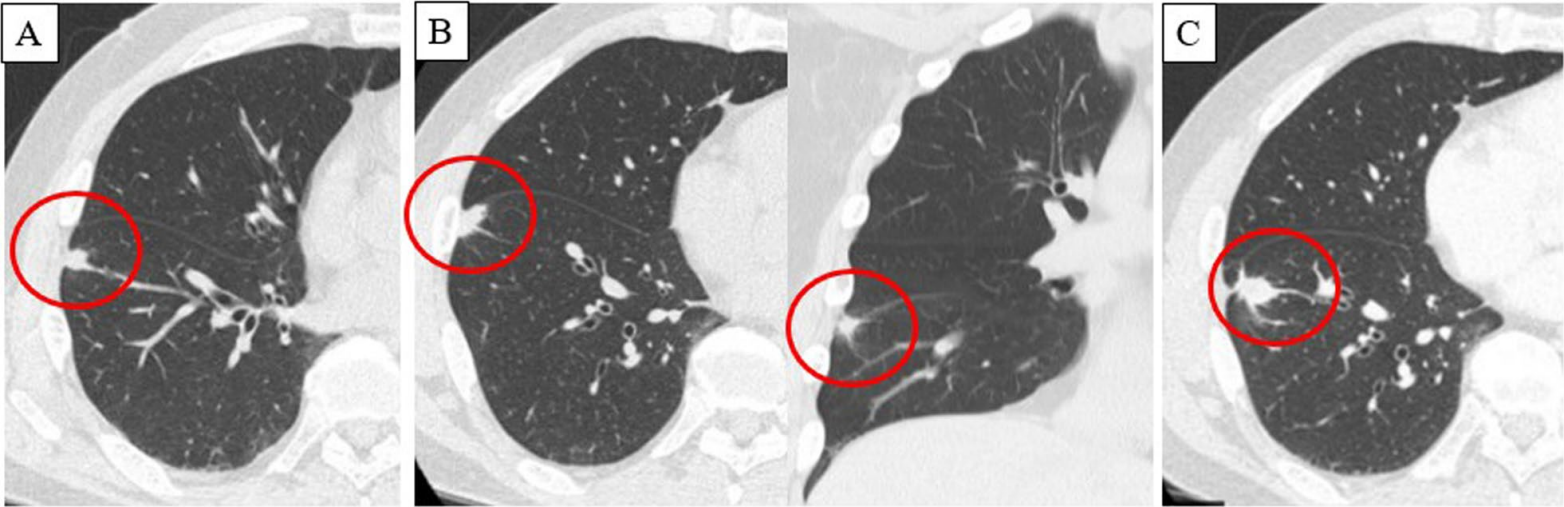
The course of the right S8 nodule on CT. Three months after the first nodal appearance (A), the right S8 nodule showed wedge-shaped nodule with linear opacity which was uncommon for a metastatic lung tumor (B). One year and three months after the nodule appeared, the size of the right S8 nodule increased to 17 mm (C)
